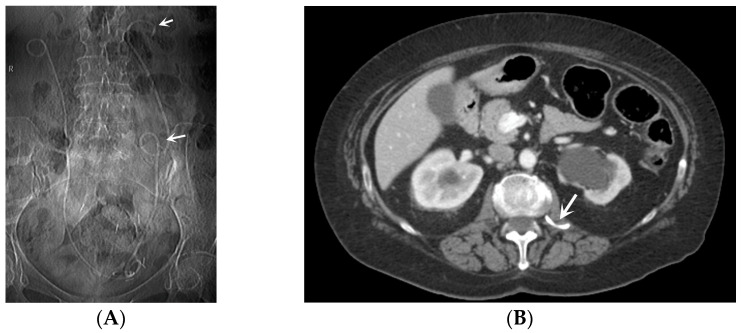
Bilateral stenting. CT scout-view (A) and axial CT scan (B). Knotted ureteral stent seen perforating the left iliac ureter and reaching the psoas muscle (arrows). Left hydronephrosis persists. The right stent is placed correctly.
